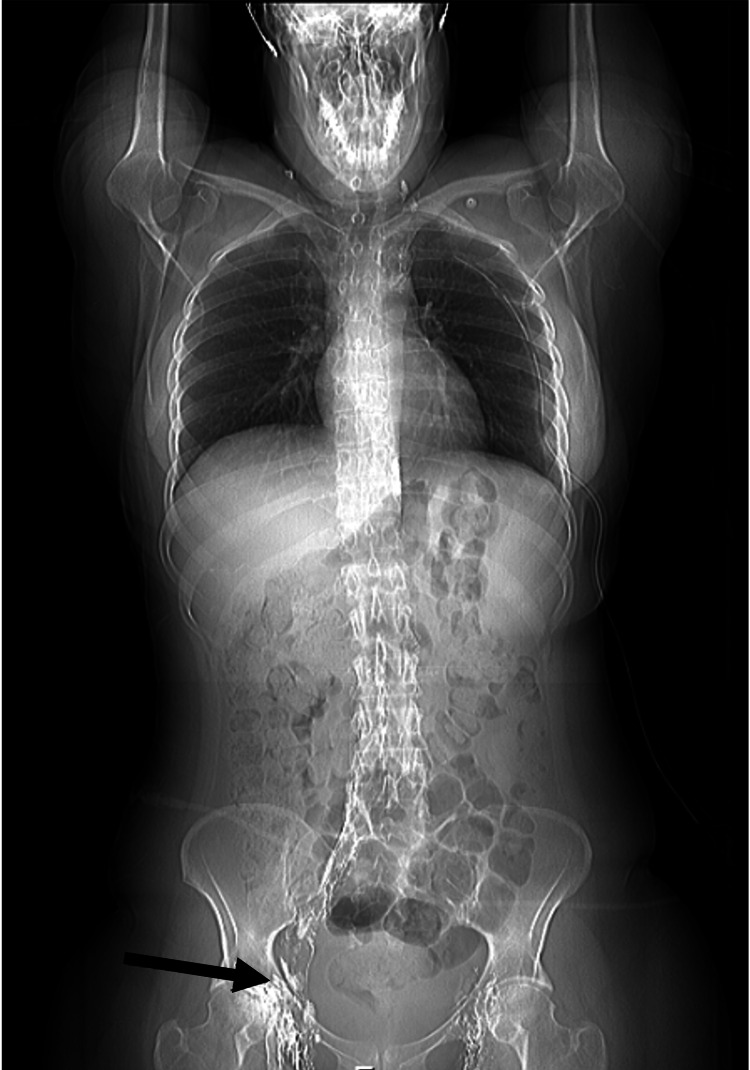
Radiographic image during intranodal lymphangiography with ultrasound-guided right inguinal lymph node approach to ethiodized oil injection (arrow).
